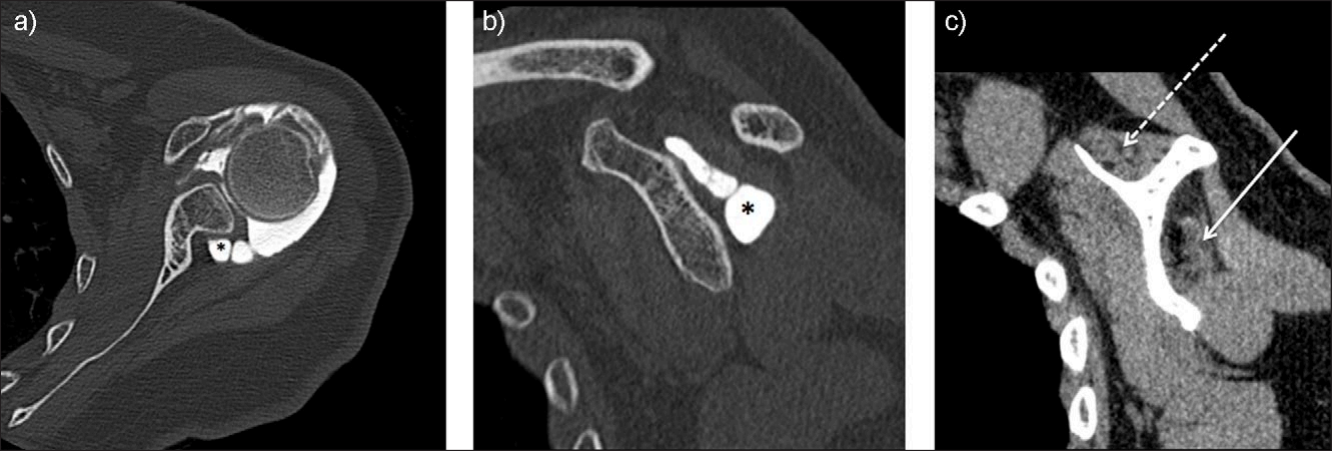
Neuropathy of suprascapular nerve by voluminous labral cyst (star) sited in spinoglenoid notch extending to supraspinatus fossa inducing a severe atrophy and fatty degeneration of infraspinatus (solid arrow) whereas the supraspinatus muscle is less involved (dashed arrow). Axial (3a = FIGURE 7 uploaded online manuscript) – sagittal (3b = FIGURE 8 uploaded online manuscript – 3c = FIGURE 9 uploaded online manuscript) CT Arthrography.
